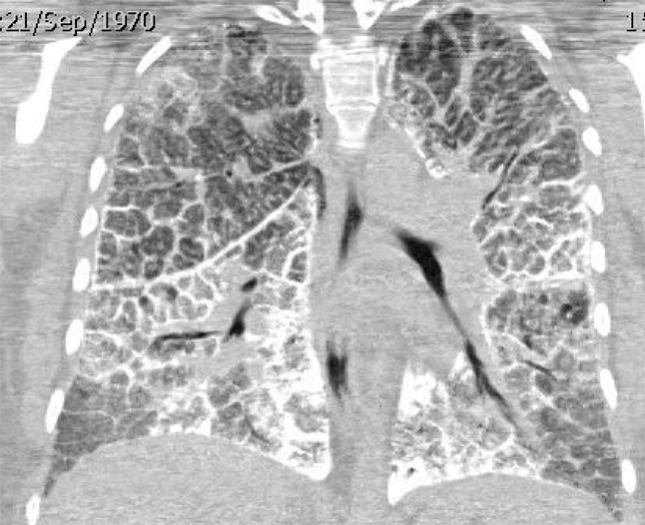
39-year-old female with reformatted coronal HRCT with diffuse interstitial and ground glass shadowing. No pleural effusion.
